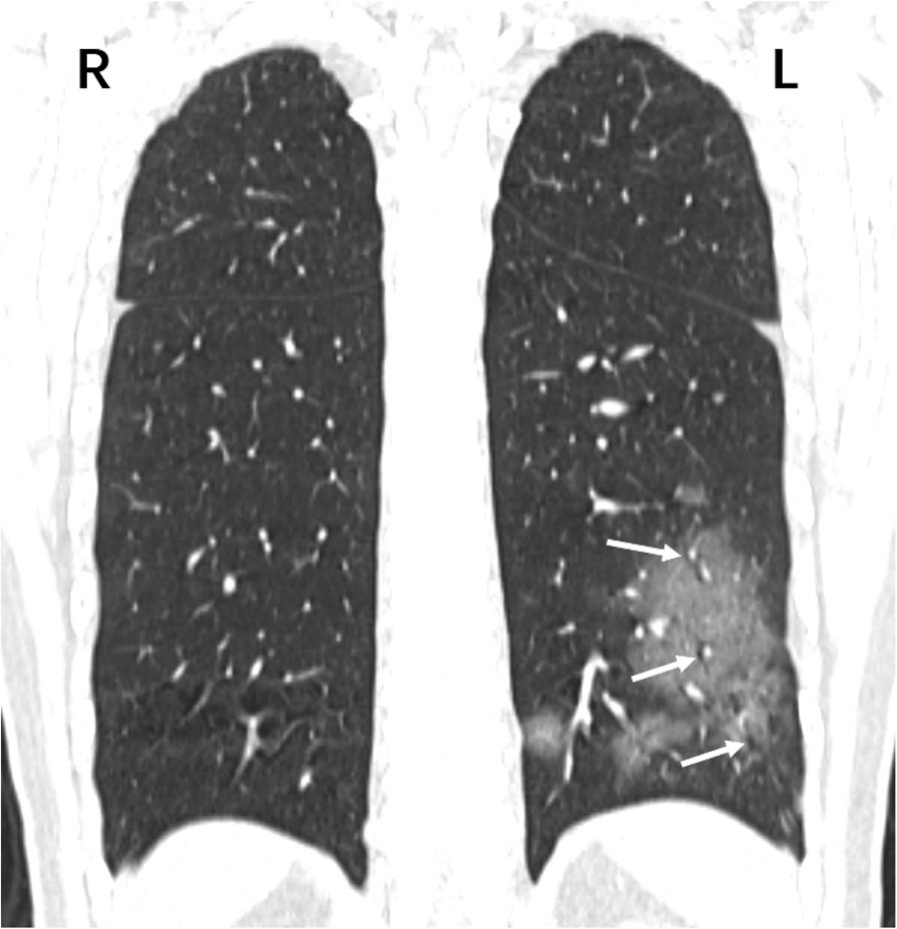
A 39-year-old female patient presenting with fever and cough, and a clear epidemiological history of contact, was admitted to our hospital. CT image shows multiple patchy GGO patterns in the lower lobes of both lungs (here, the reconstructed coronal CT image only shows lesions in the left lung). Long white arrows indicate multiple air-filled bronchi inside the lesions. NAT confirmed that the patient was infected with COVID-19. R = Right, L = Left, GGO = Ground-glass opacity, NAT = Nucleic acid test
